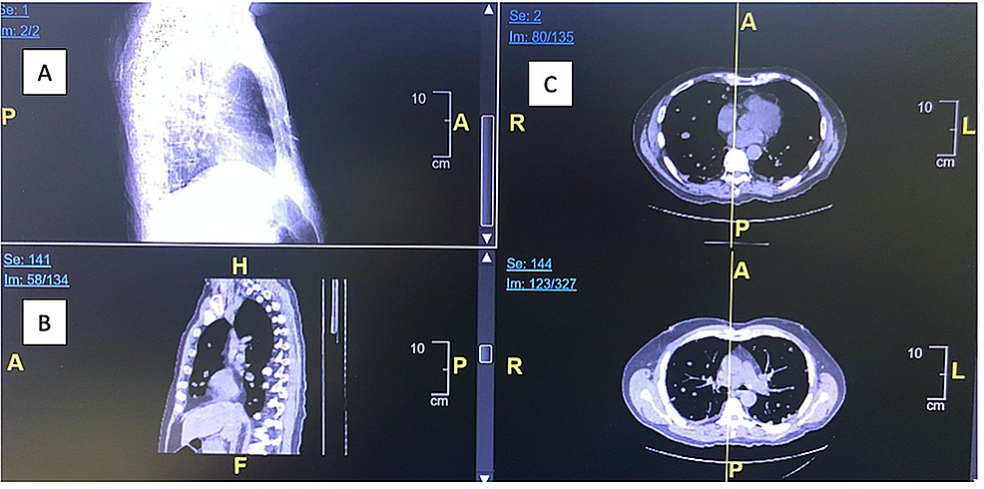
(A-C) CT imaging indicating diffuse metastatic disease involving the liver and lungs, diffuse moderate bulky peritoneal adenopathy along the mesenteric root and liver hilar region, and a small amount of free intraperitoneal fluid.
